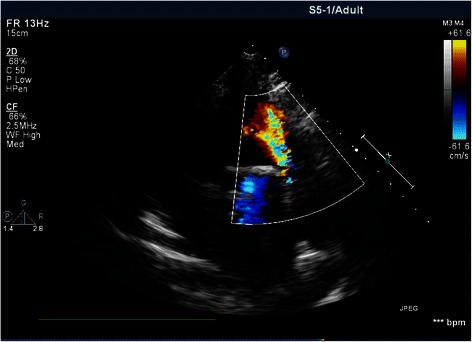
Echocardiogram showing moderate to severe regurgitation within the pulmonary valve.
